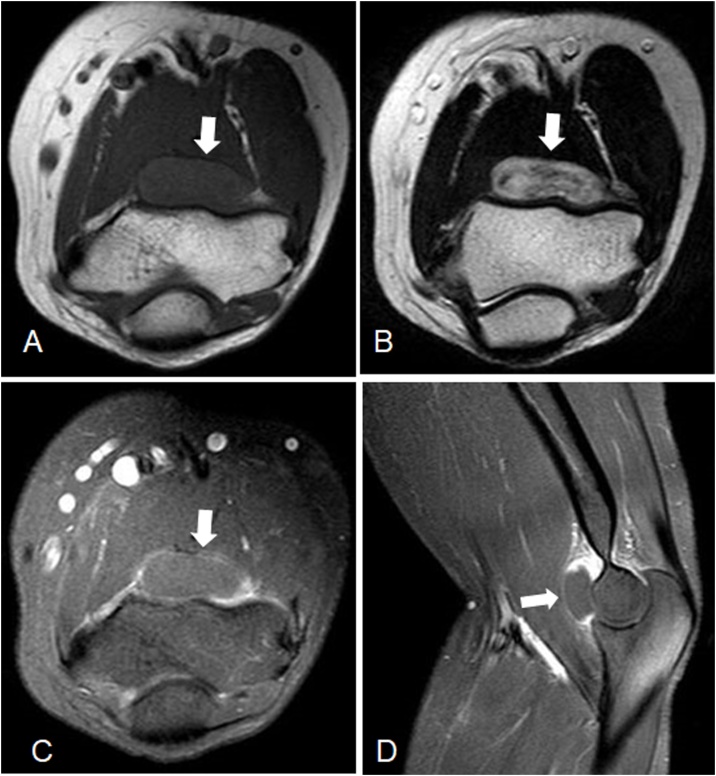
Preoperative axial T1-weighted (A) and axial T2-weighted (B) magnetic resonance (MR) images. Contrast-enhanced T1-weighted axial (C) and sagittal (D) MR images.
